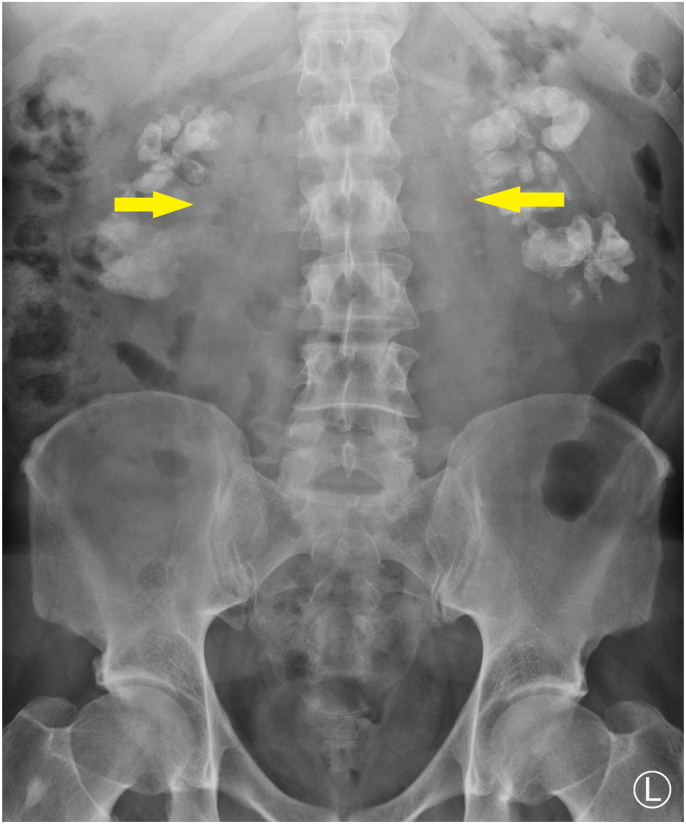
Post-operative plain film of the abdomen. Decreased stone burden in each renal pelvis is demonstrated.
